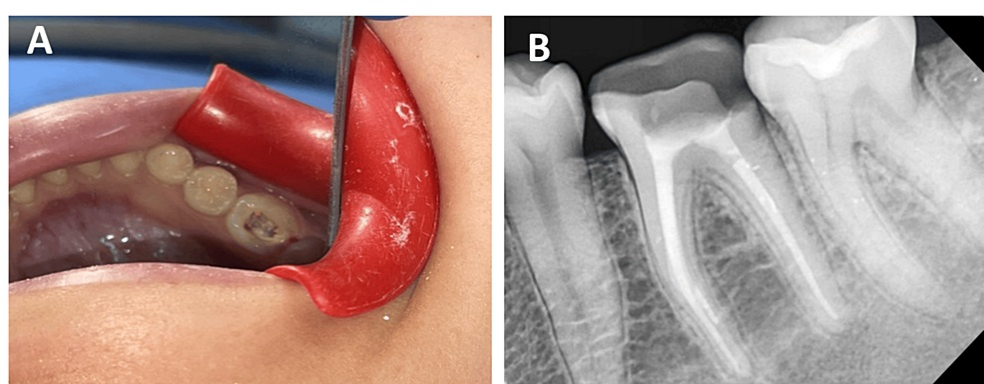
Evaluation of the tooth after endodontic treatment: (A) Clinical examination. (B) Radiographic examination
